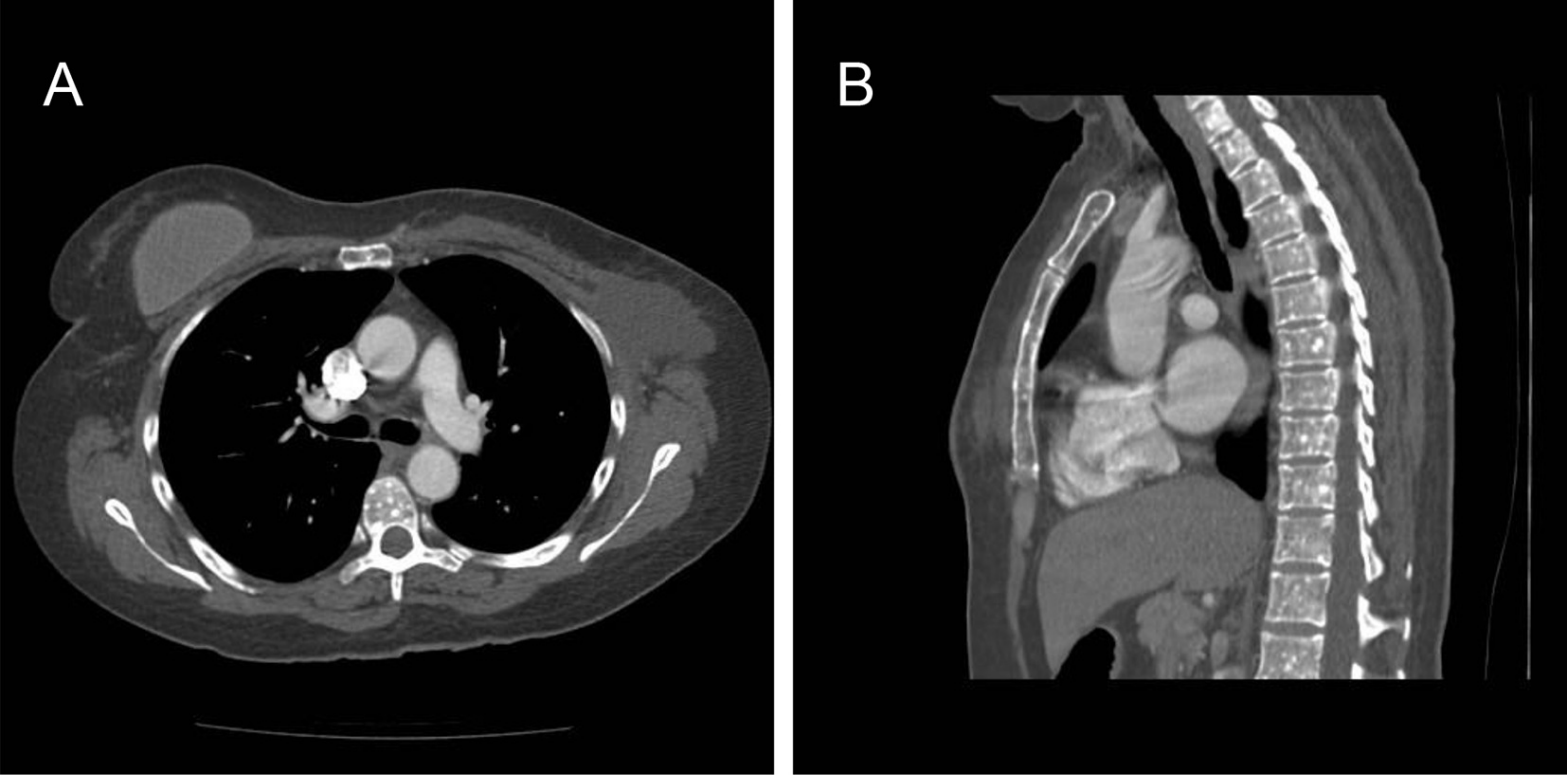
CT scan of a 50-year-old female with node-positive breast cancer (Case 2). (a) Axial CT image of the thorax showing multiple, small, rounded sclerotic foci in the T7 vertebral body. (b) Sagittal CT image showing multiple, small, rounded, sclerotic lesions throughout the vertebra and sternum.
